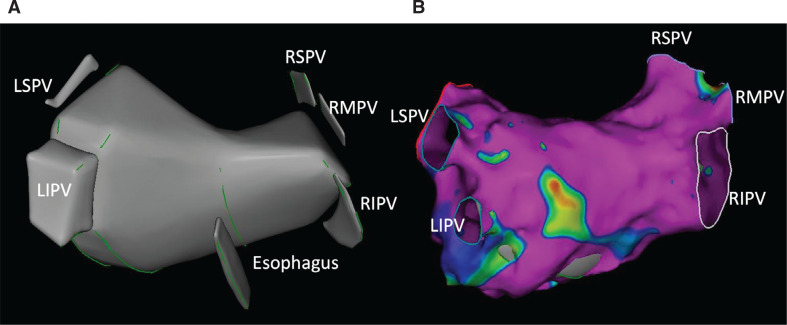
(A) An intracardiac echocardiography shell of the left atrium and its structures, which was used as a reference to construct (B) an electroanatomical map using the multipolar catheter after the transseptal puncture. *Abbreviations:* LIPV, left inferior pulmonary vein; LSPV, left superior pulmonary vein; RIPV, right inferior pulmonary vein; RMPV, right middle pulmonary vein; RSPV, right superior pulmonary vein.
